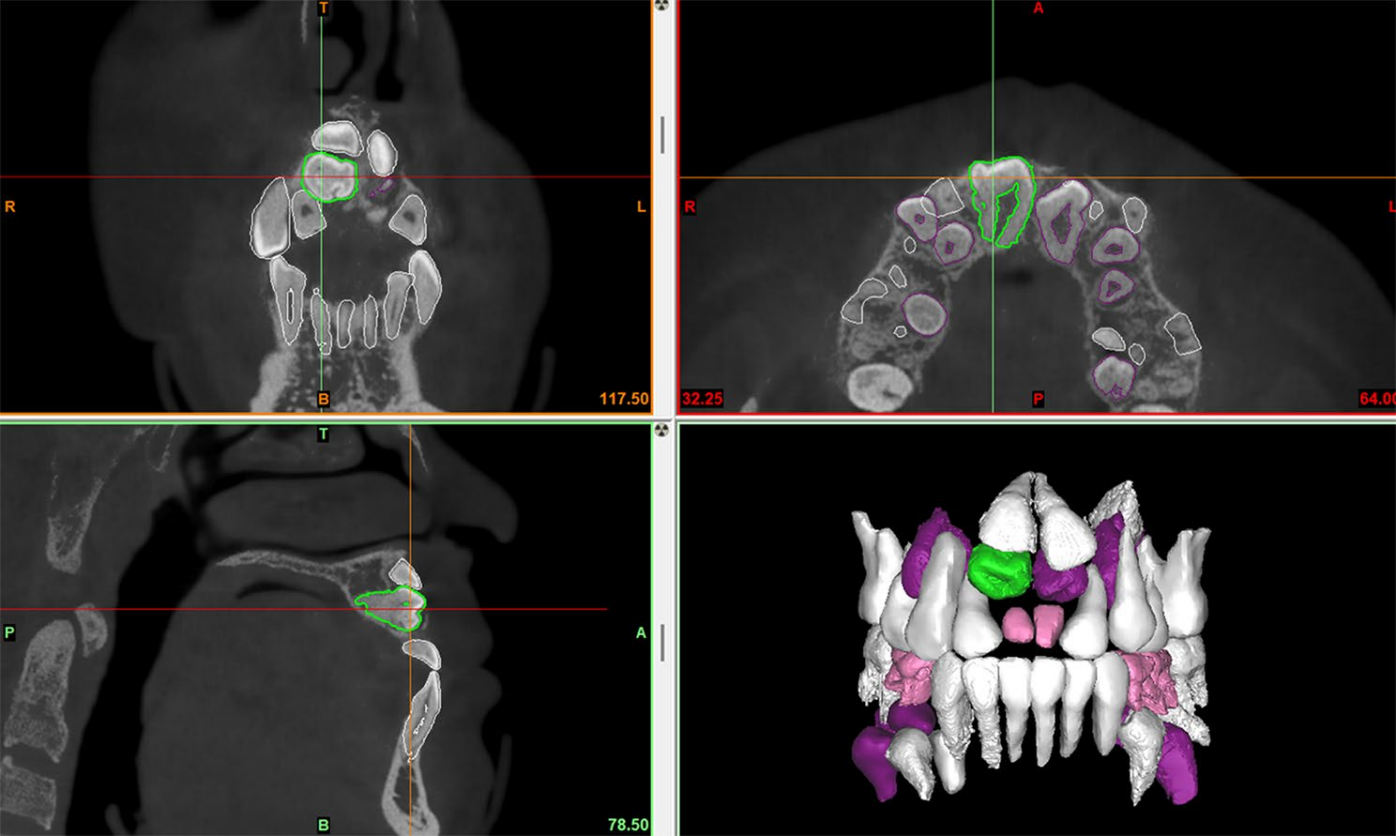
A coronal supernumerary tooth located in right anterior maxillary region (sagittal, coronal, axial sections of CBCT images and 3D image reconstruction)
